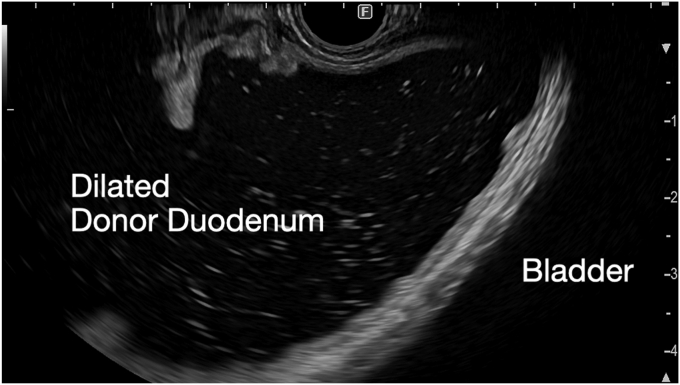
Representative trans-sigmoidal endosonographic image of the dilated donor duodenum adjacent to the bladder.
